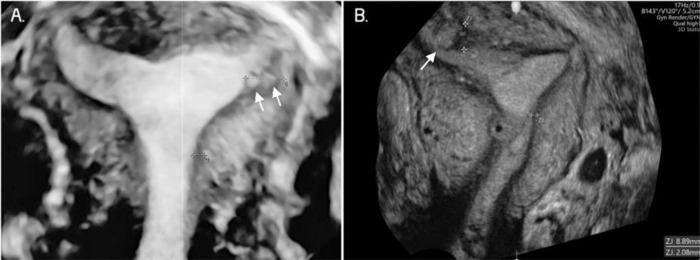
Three-dimensional ultrasound imaging of a uterus with adenomyosis in the coronal plane. A. Protrusions of the endometrium into the junctional zone (JZ) (arrows). B. Junctional zone thickened, interrupted, and infiltrated by subendometrial echogenic nodules (buds) (arrows). In this image, the JZ difference is 6.8 mm.
